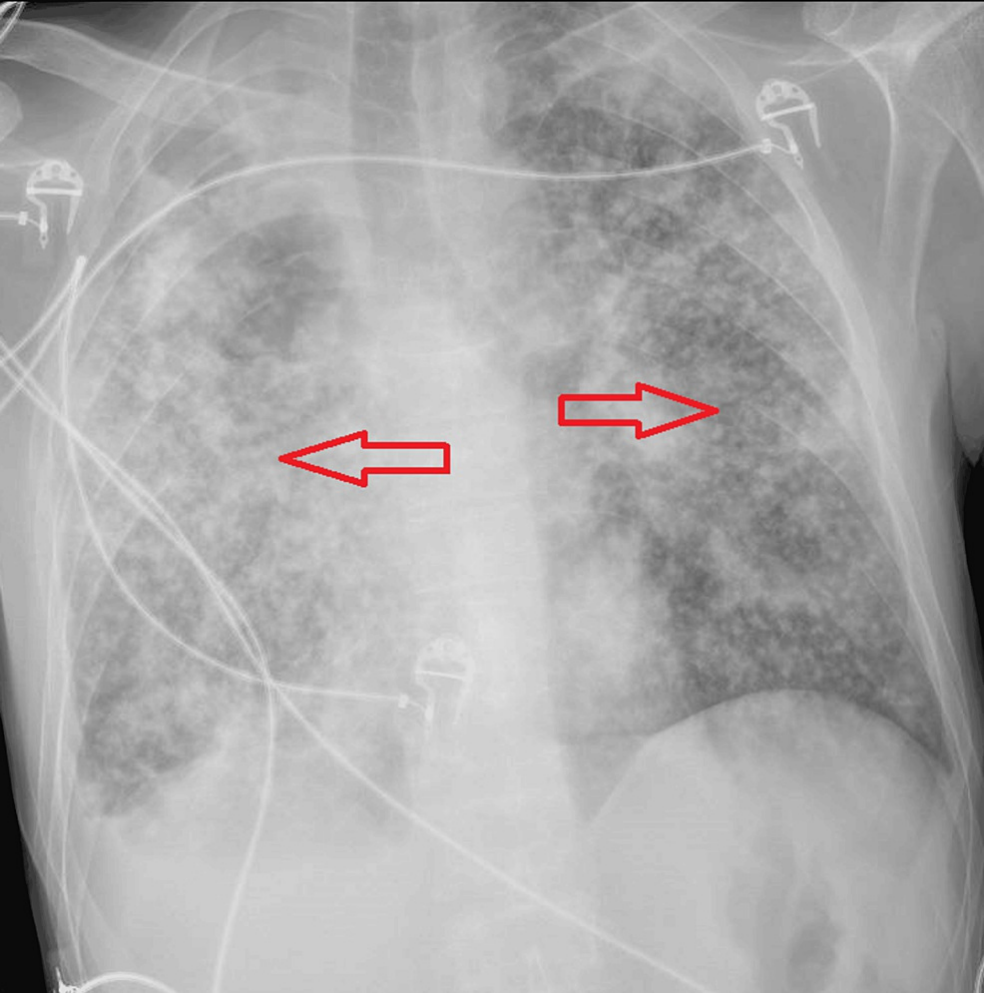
Diffuse tuberculosis miliary pattern on x-ray (marked with red arrows)
